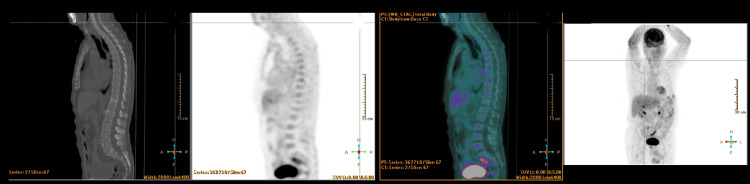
PET-18FDG (Positron Emission Tomography with 18F-Fluorodeoxyglucose) In the lung parenchyma, a small nodular formation in the upper left lobe, apparently calcified, likely related to a granuloma is noticeable. No clearly suspicious hypermetabolic lymph nodes are seen. Liver, spleen, adrenal glands, and pancreas show no suspicious hypermetabolic changes. Increased uptake of 18F-FDG is noted in the intestinal loops, likely of physiological nature. There is increased uptake of 18F-FDG in the lateral aspect of the 7th, 8th, and 9th left rib arcs, associated with traces of fracture. The remaining skeleton shows diffuse uptake of the radiopharmaceutical, with mild intensity and heterogeneous distribution. No other significant findings were noted. There were no functional changes suggestive of high-grade malignant neoplastic lesions with metabolic activity.
